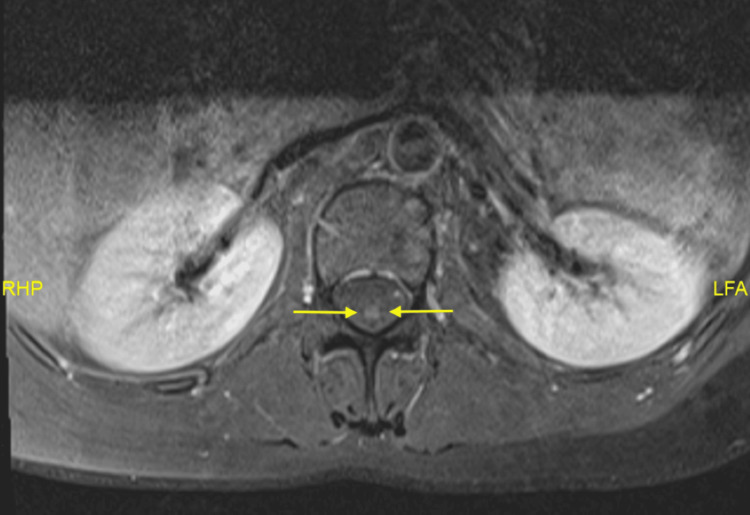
MRI lumbosacral with gadolinium (T1 sequence) shows enhancement of the central canal (yellow arrow)
